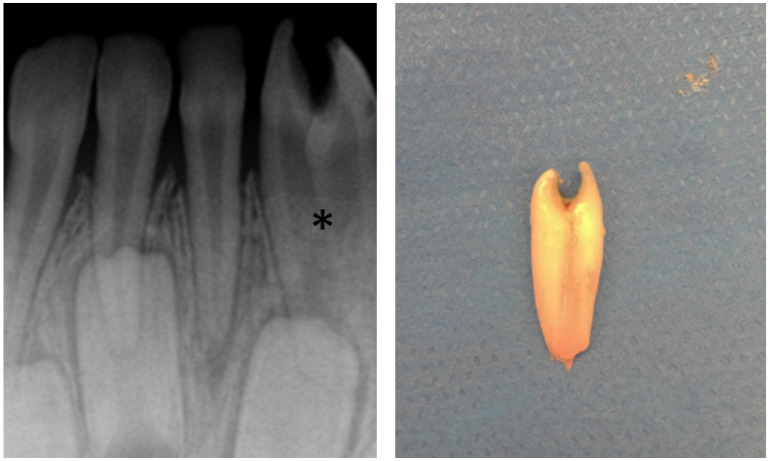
Geminated primary lower lateral incisor shown radiographically (*) and photographically following extraction. When counted as one tooth, the patient had the appropriate number of teeth, confirming this was a geminated tooth as opposed to the fusion of two teeth.
